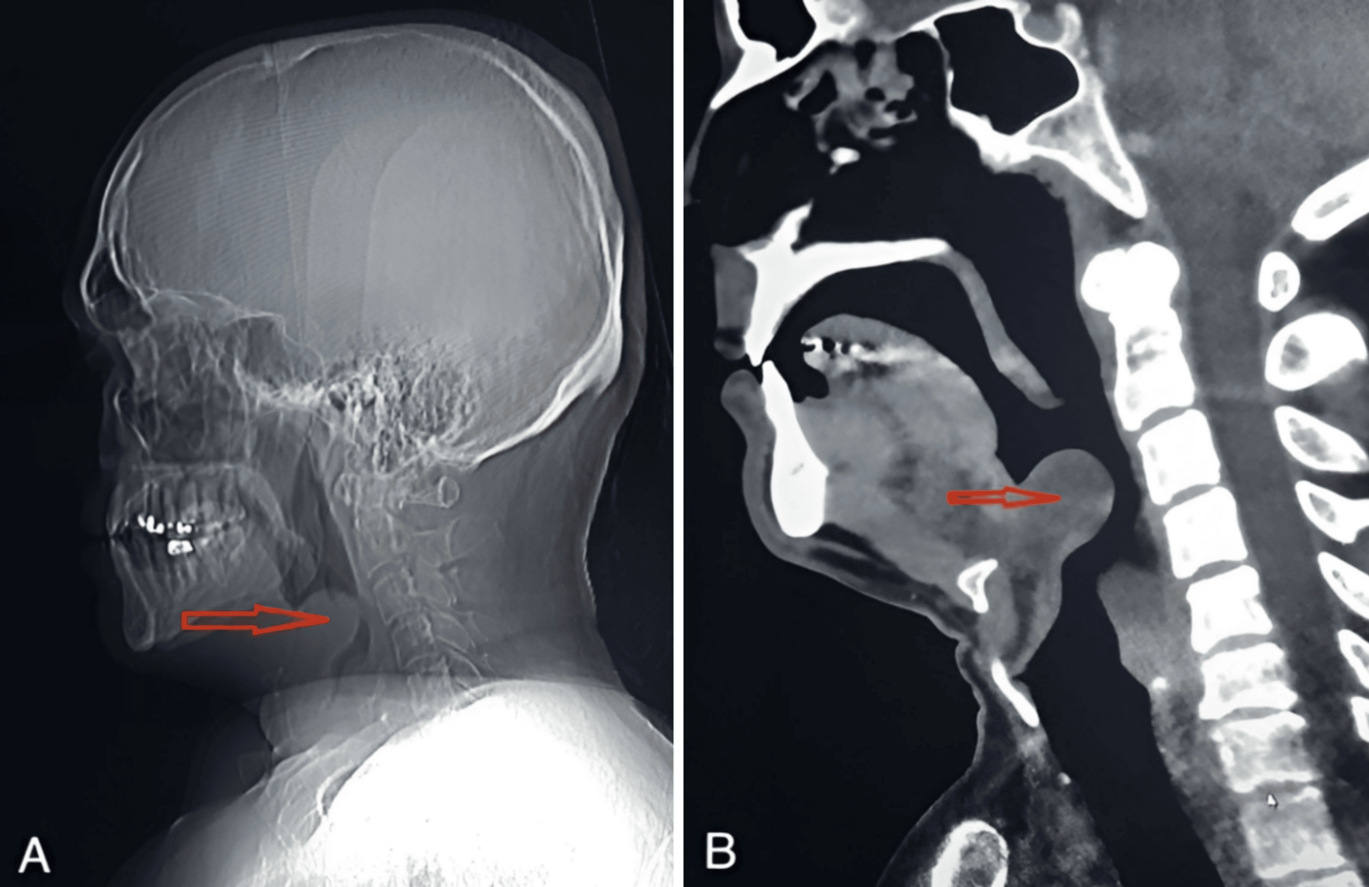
X-ray (A) and CT (B) scans of Patient X indicating inflammation of the epiglottis (red arrows) causing presentation of symptoms. This is commonly known as the “thumbprint sign”.
